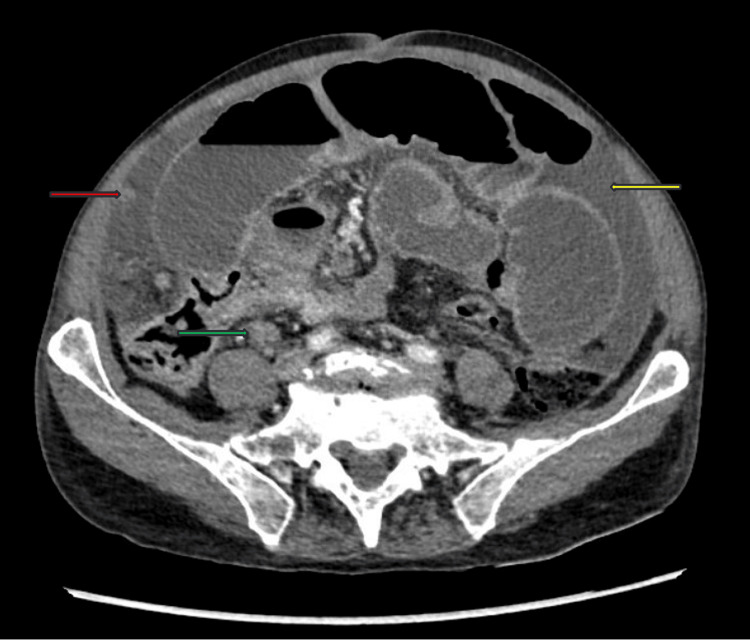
Abdominal CT scan on presentation Cross-sectional computed tomography (CT) image of the abdomen showing large-volume ascites (yellow arrow), mesenteric adenopathy in the right lower quadrant (green), and peritoneal thickening and nodularity (red). No abnormalities appreciated in intra-abdominal and intra-pelvic organs. Contiguous axial 1.25 mm images were obtained from the lung bases to the greater trochanters with 100 cc Isovue 370 intravenous contrast in the portal venous phase. Coronal, sagittal, and 3D maximum intensity projection (MIP) reconstructions were obtained.
